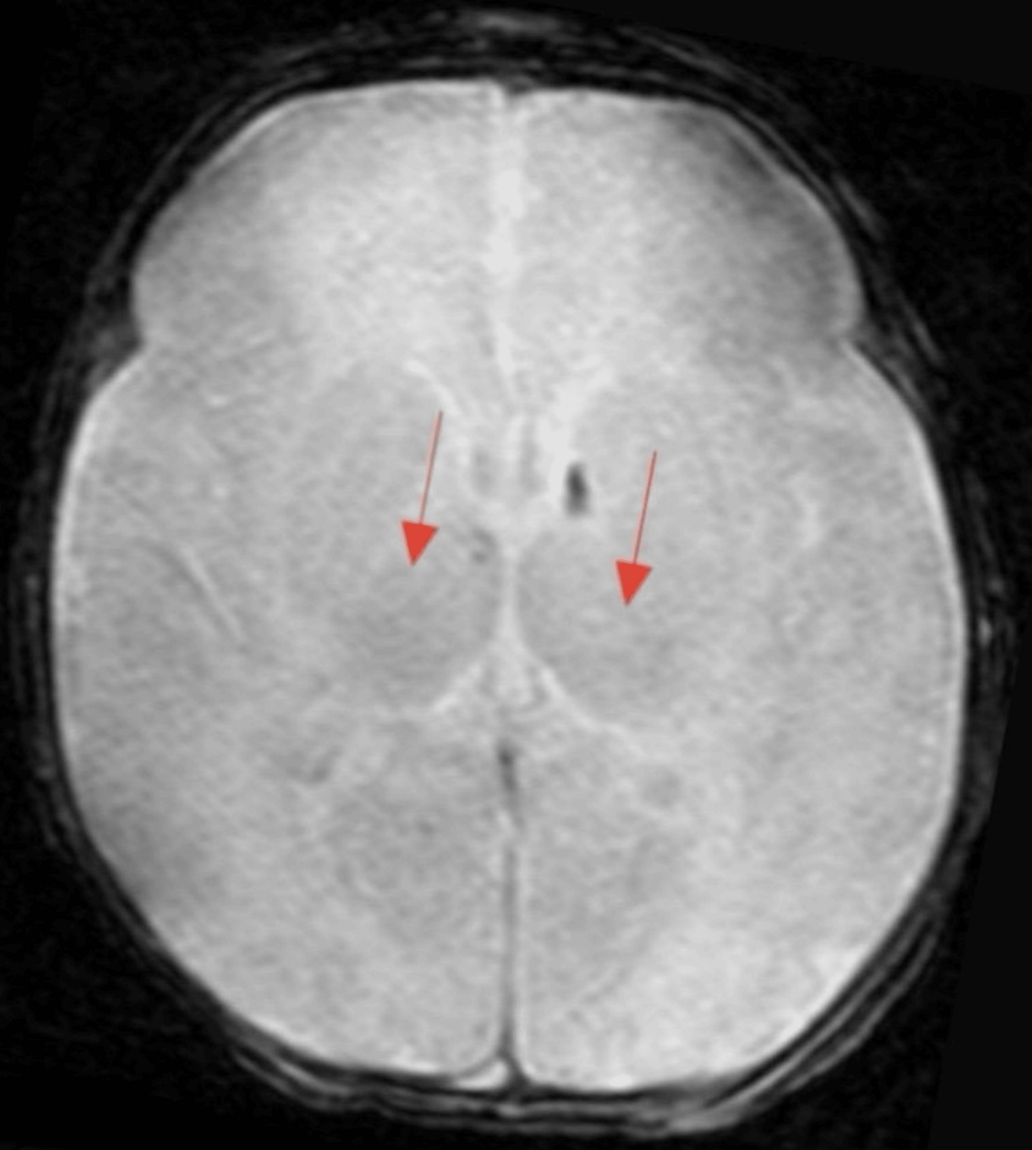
Brain MRI (axial view) showing T1 intraventricular hemorrhage (arrows)
